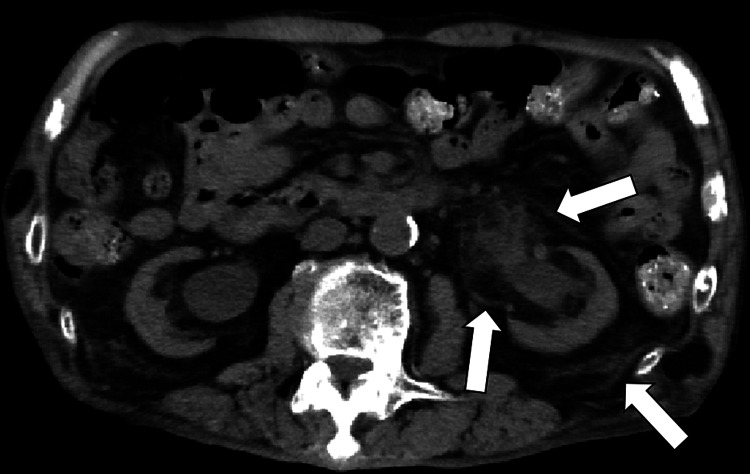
A computed tomography scan of the abdomen showing increased fat tissue density around the left kidney (white arrows)
